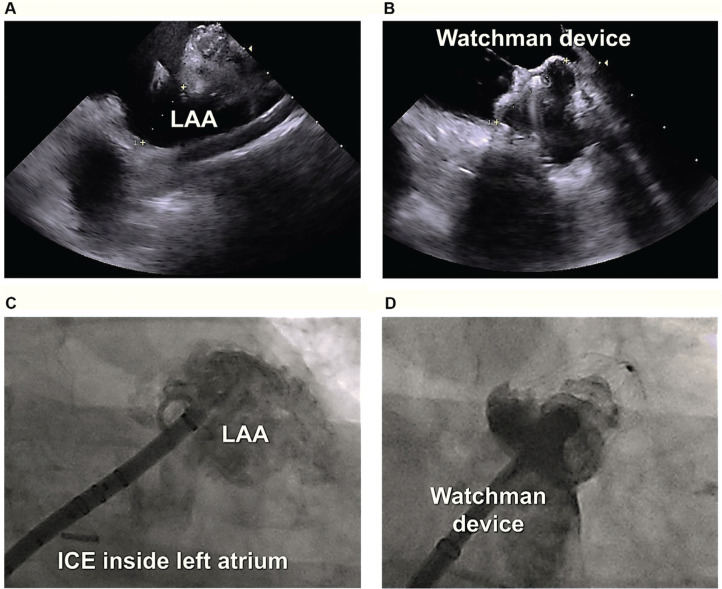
Left atrial appendage (LAA) occlusion using a Watchman™ device guided by ICE. Shown are the LAA size measurements and morphology as demonstrated by ICE from inside the left atrium (A) and angiography (B). Endocardial LAA occlusion was subsequently performed using a 27-mm Watchman FLX™ device guided by ICE imaging (C) and contrast angiography (D). Abbreviations: ICE, intracardiac echocardiography; LAA, left atrial appendage.
